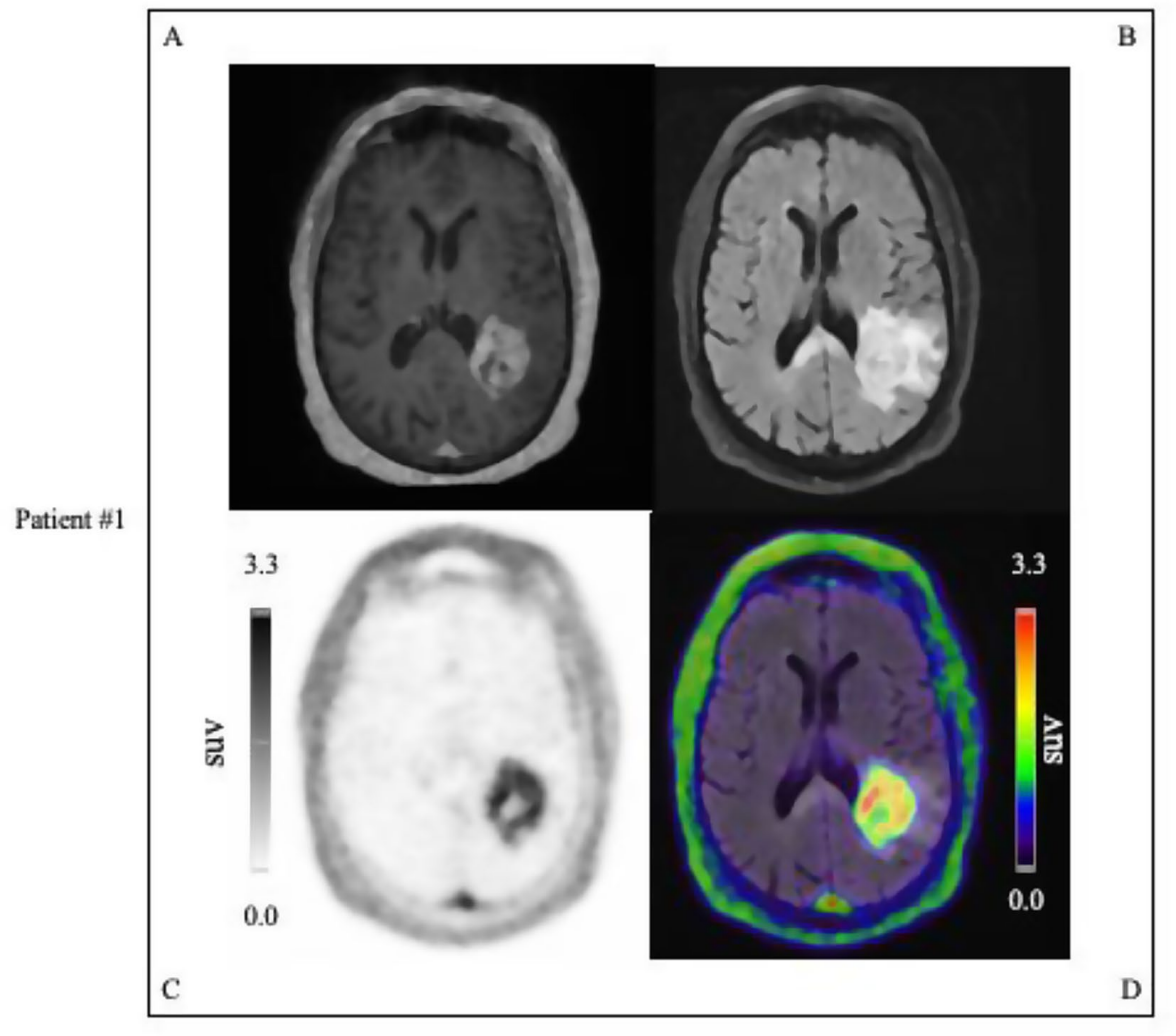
Examples of uPAR PET/MRI performed on patient with glioblastoma, IDH-wildtype, WHO Grade 4 (MGMT non-methylated) in the temporoparietal lobe with tumor SUVmax 3.3: #A T1W MPRAGE MRI with gadolinium contrast, #B T2W FLAIR MRI image, #C uPAR-PET image, #D Merged T2W FLAIR MRI, and uPAR-PET image. Color scale from 0 to tumor SUVmax value of 3.3
